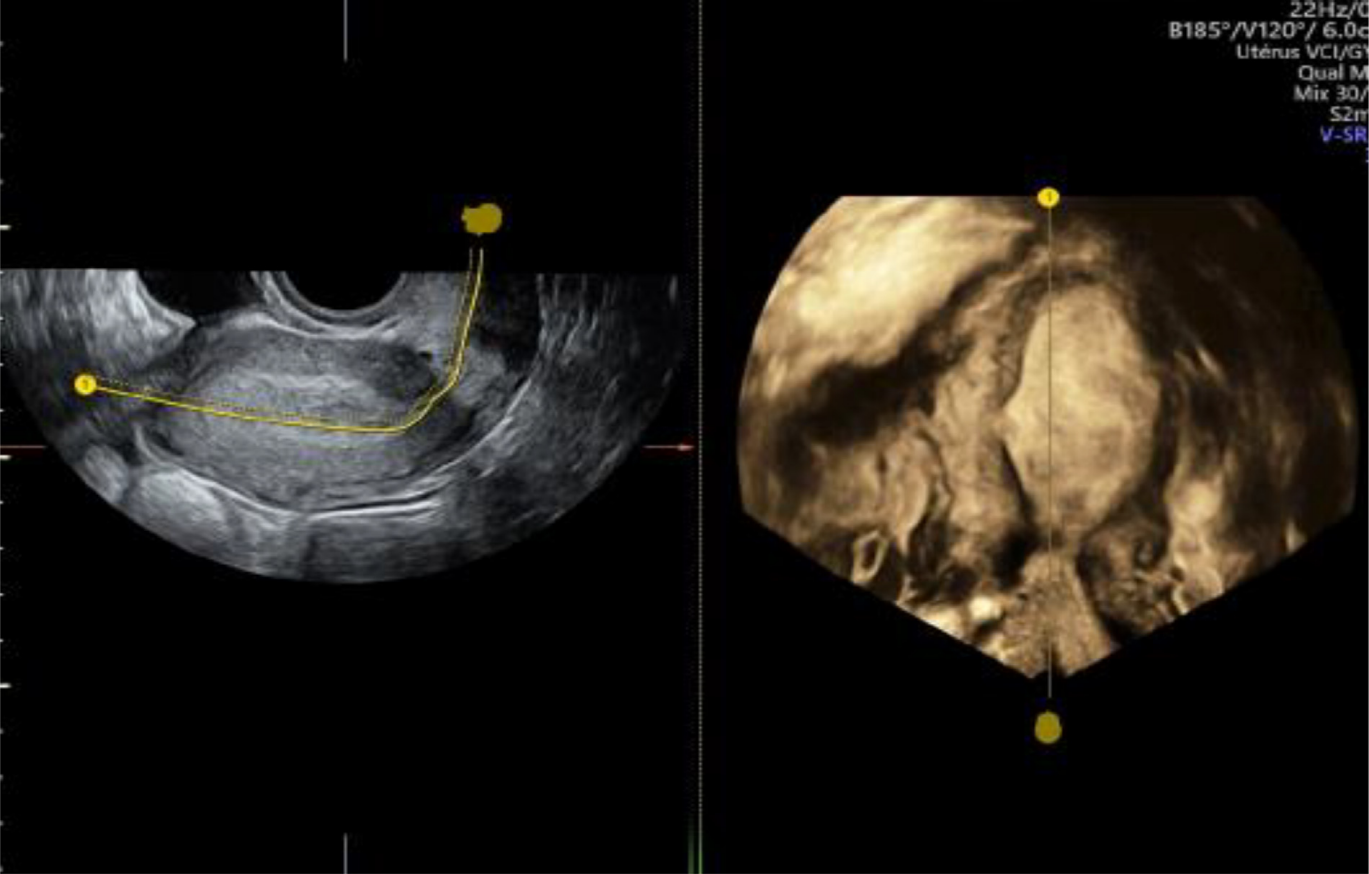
Enovaginal ultrasound 3D empty unicorn uterus.
